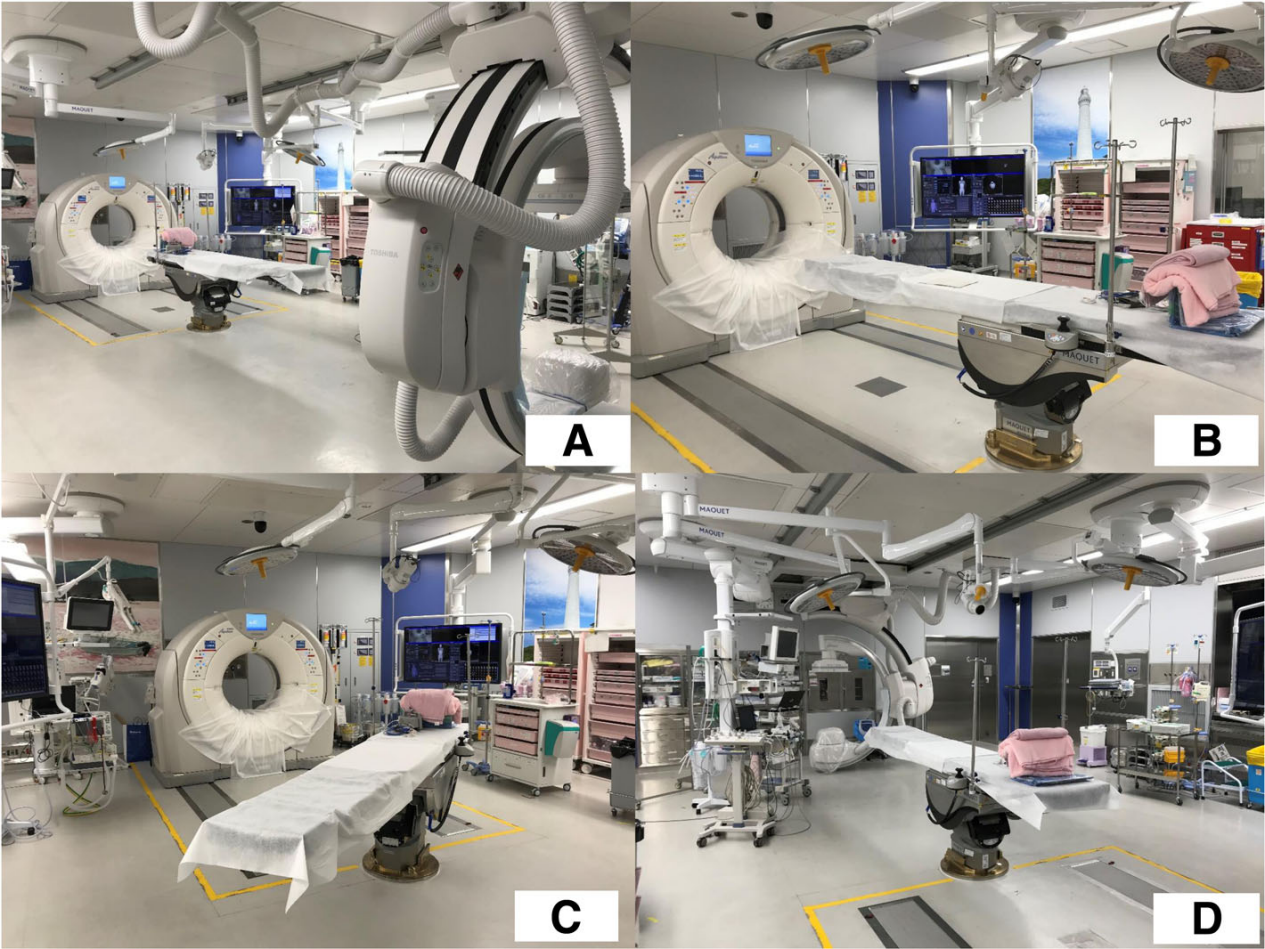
a shows that C-arm and CT gantry are located at the opposite side. b shows CT mode in which primary survey and CT are performed. c shows rotating patient table. d shows intervention mode in which surgeries and interventional radiology are performed after rotating the patient table
